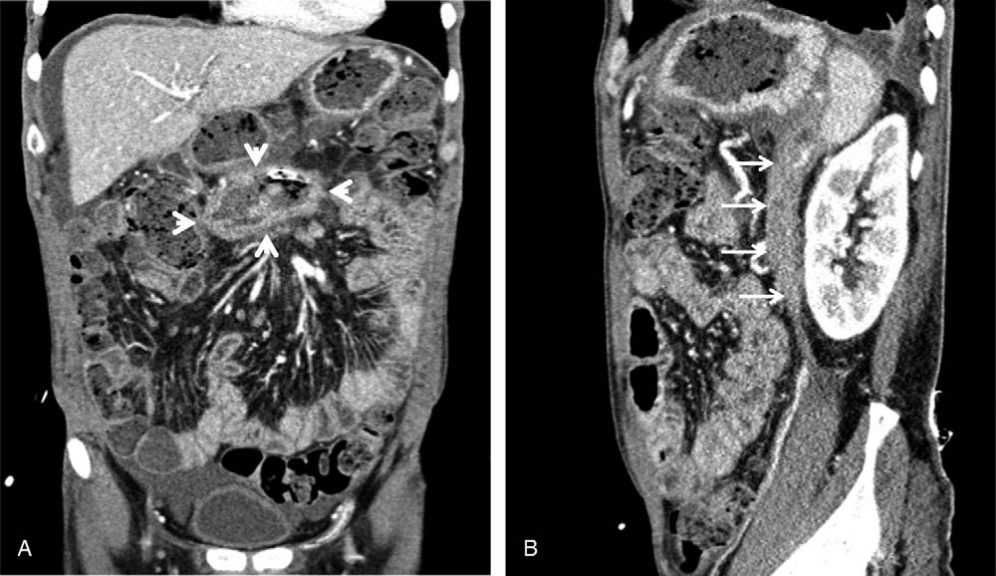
Abdominal CT showing near complete resolution of the infected WON, except around the pancreatic head. A: A coronal CT scan showing the WON with necrotic tissues and fluids still present around the head of the pancreas (white arrowhead). B: A sagittal CT scan showing near complete resolution of the gas and necrotic tissues in the left anterior pararenal space (white arrow). CT = computed tomography, WON = walled-off necrosis.
